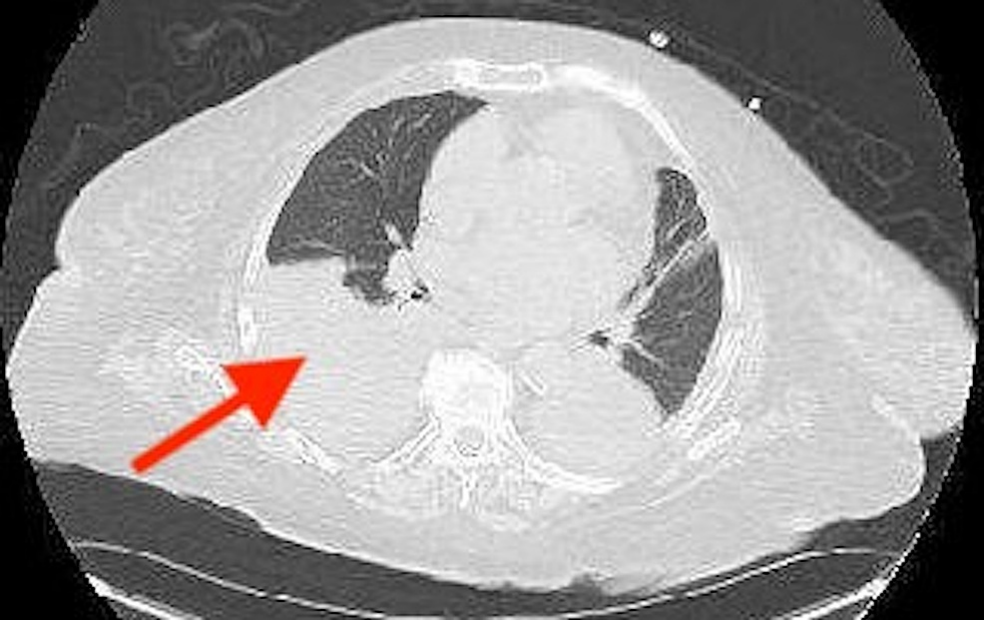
CT chest without contrast axial view showing moderate right pleural effusion (red arrow).
